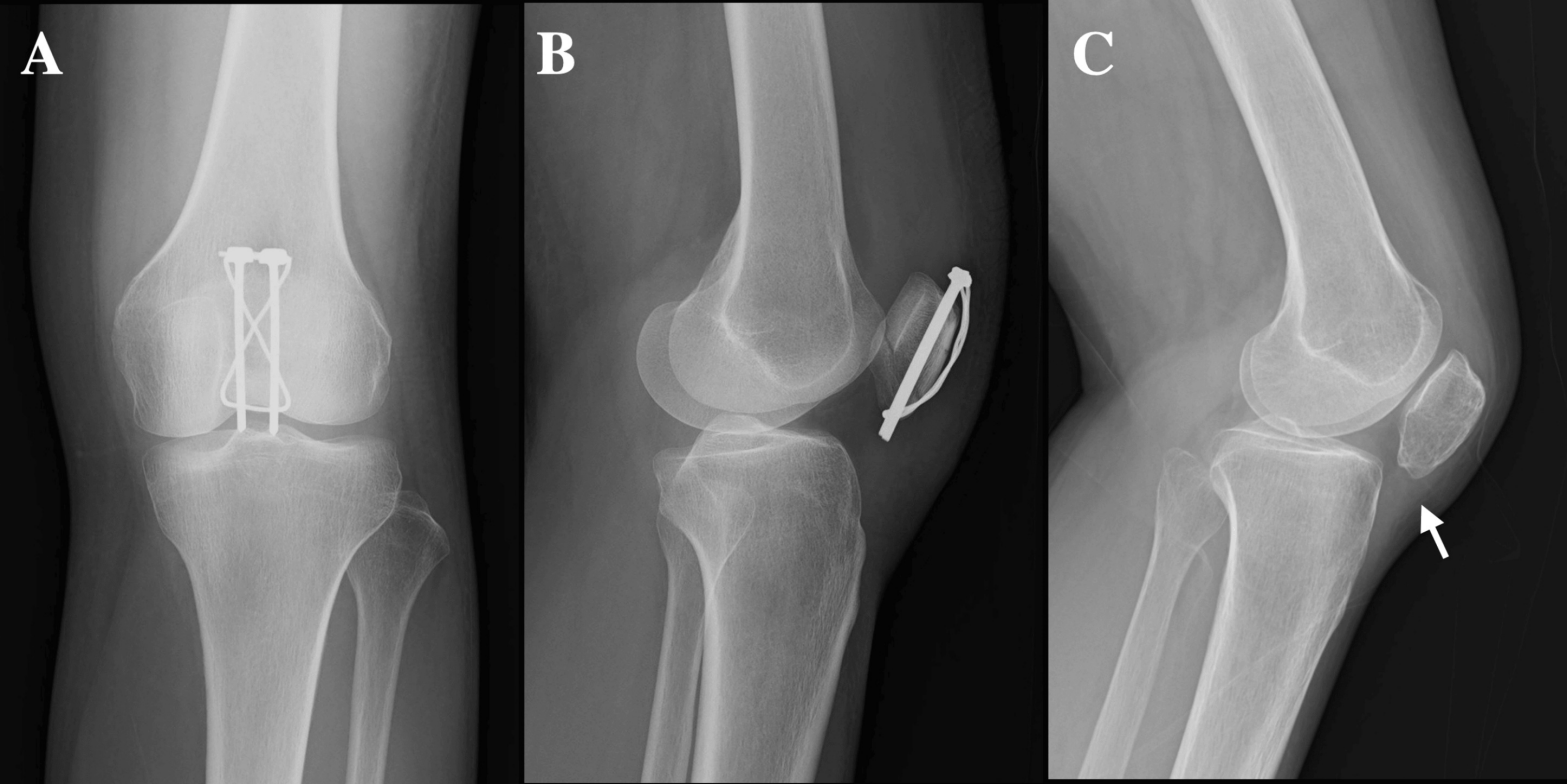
Preoperative radiographs Tension band wiring was performed for a patellar fracture (AO/OTA Type A1) at another institution (A, B). After implant removal, the patient was found to have marked patella baja (C, arrow).
